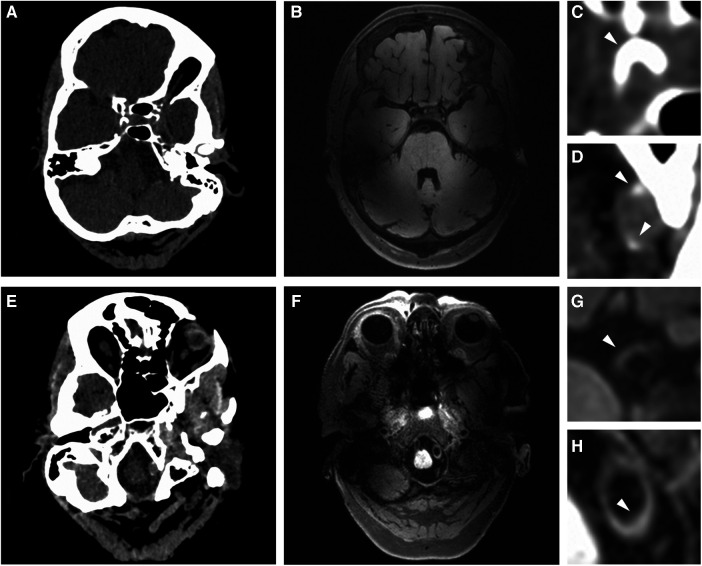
Imaging proxies of intracranial arterial disease. (A,E) Example of intracranial artery calcification on axial non-contrast head CT. (B,F) Example of vessel wall lesions on 7 T MRI. Zoomed images show: (C) a thick arterial calcification in the right ICA segment; (D) two calcified spots in the left VA segment; (G) a vessel wall lesion in the right ICA; (H) vessel wall lesion affecting a portion of the left VA.
